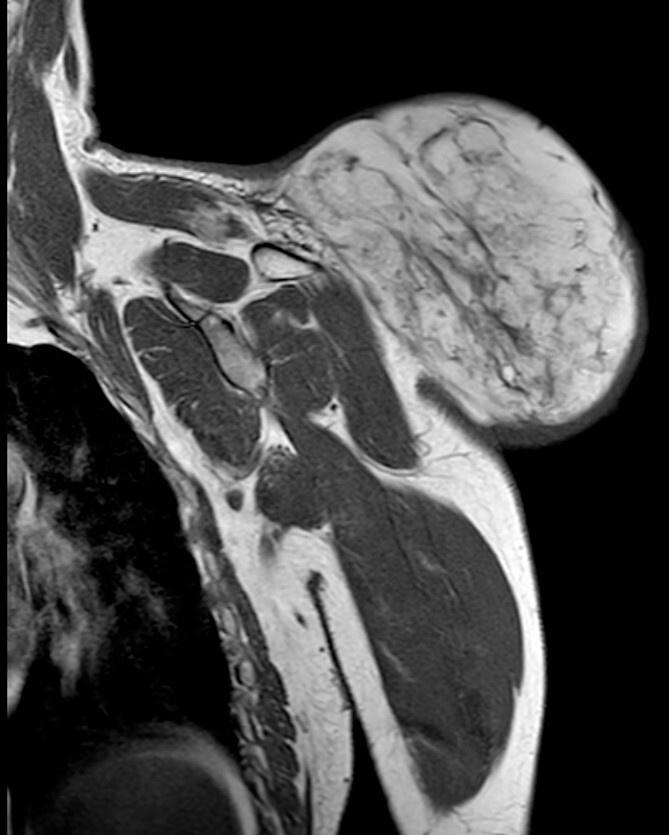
Magnetic resonance imaging T1: polylobulated lesion with multiple septa separating different areas of fat tissue.
